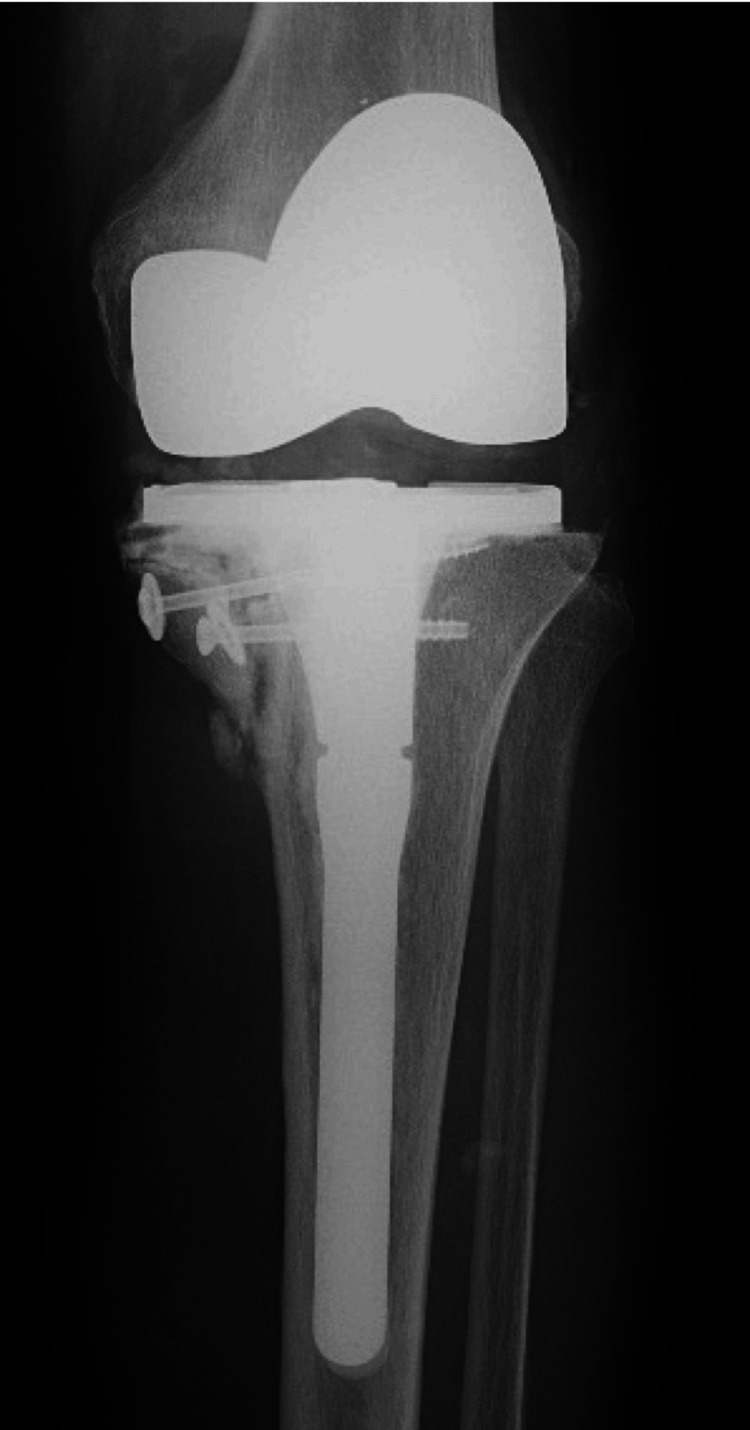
Immediate postoperative radiography of TKA (AP view) TKA: total knee arthroplasty, AP: anteroposterior.
